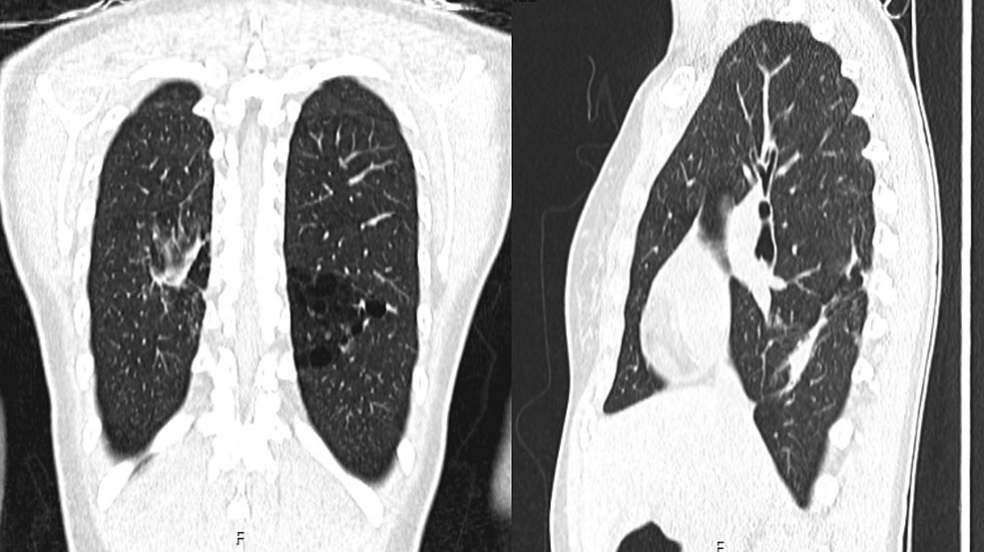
Follow-up chest CT scan three months after treatment completion
